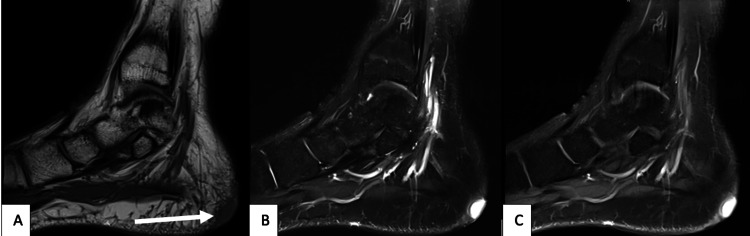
MRI of the patient Sagittal non-contrast T1 image (a) of the medial aspect of the foot showing low signal skin lesion in the posterior heel region (arrow); T2 image with fat suppression (b) showing bright signal lesion in the skin of the posterior foot, with the post-contrast image (c) redemonstrating intense and relatively homogenous enhancement of the lesion MRI: magnetic resonance imaging
